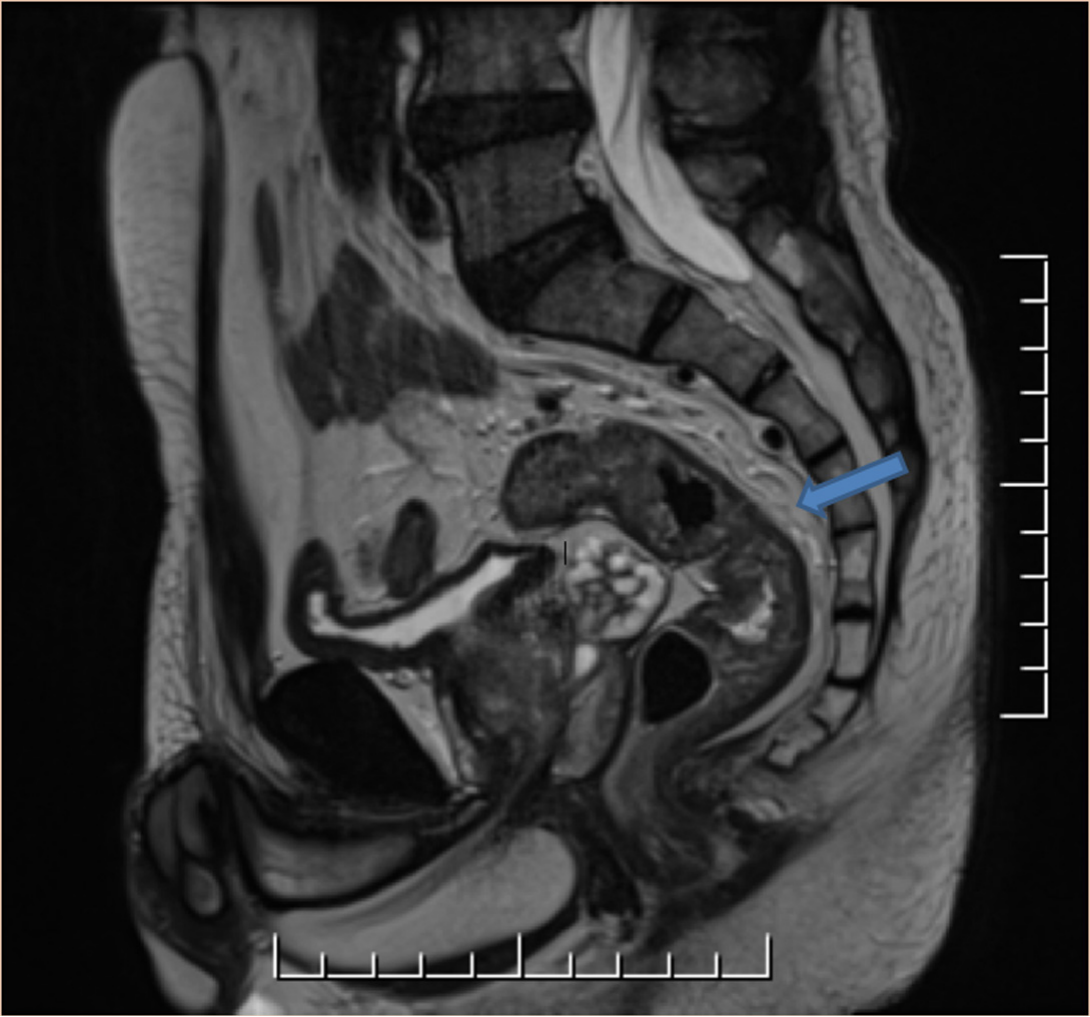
Sagittal MRI pelvis demonstrating T2/T3 rectosigmoid mass.
